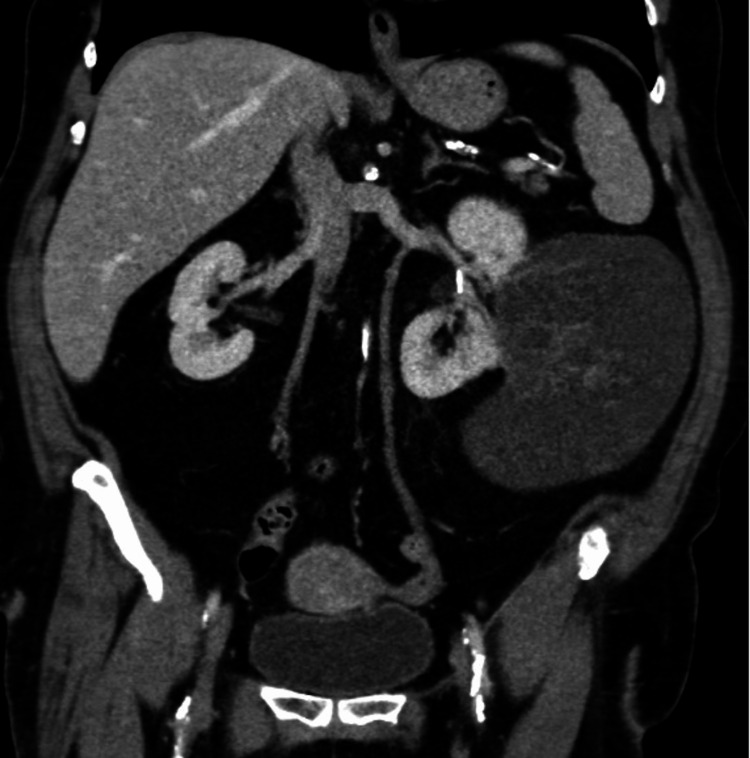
Unenhanced coronal CT scan demonstrating a large heterogenous left exophytic renal mass CT: computed tomography
